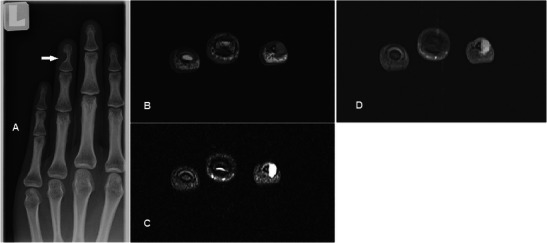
Glomus tumour in a 30-year-old female with a painful lump at the tip of the left ring finger. (a) Plain radiograph shows well-defined scalloping along the ulnar aspect of the left ring finger distal phalanx (arrow). (b) T1w sequence revealed a smooth, well-circumscribed subcutaneous lesion causing bony pressure erosion at the tip of the finger of intermediate signal. (c) T2w-FS sequence shows the lesion to be extremely and homogeneously hyperintense. (d) Intense and homogeneous enhancement is seen
